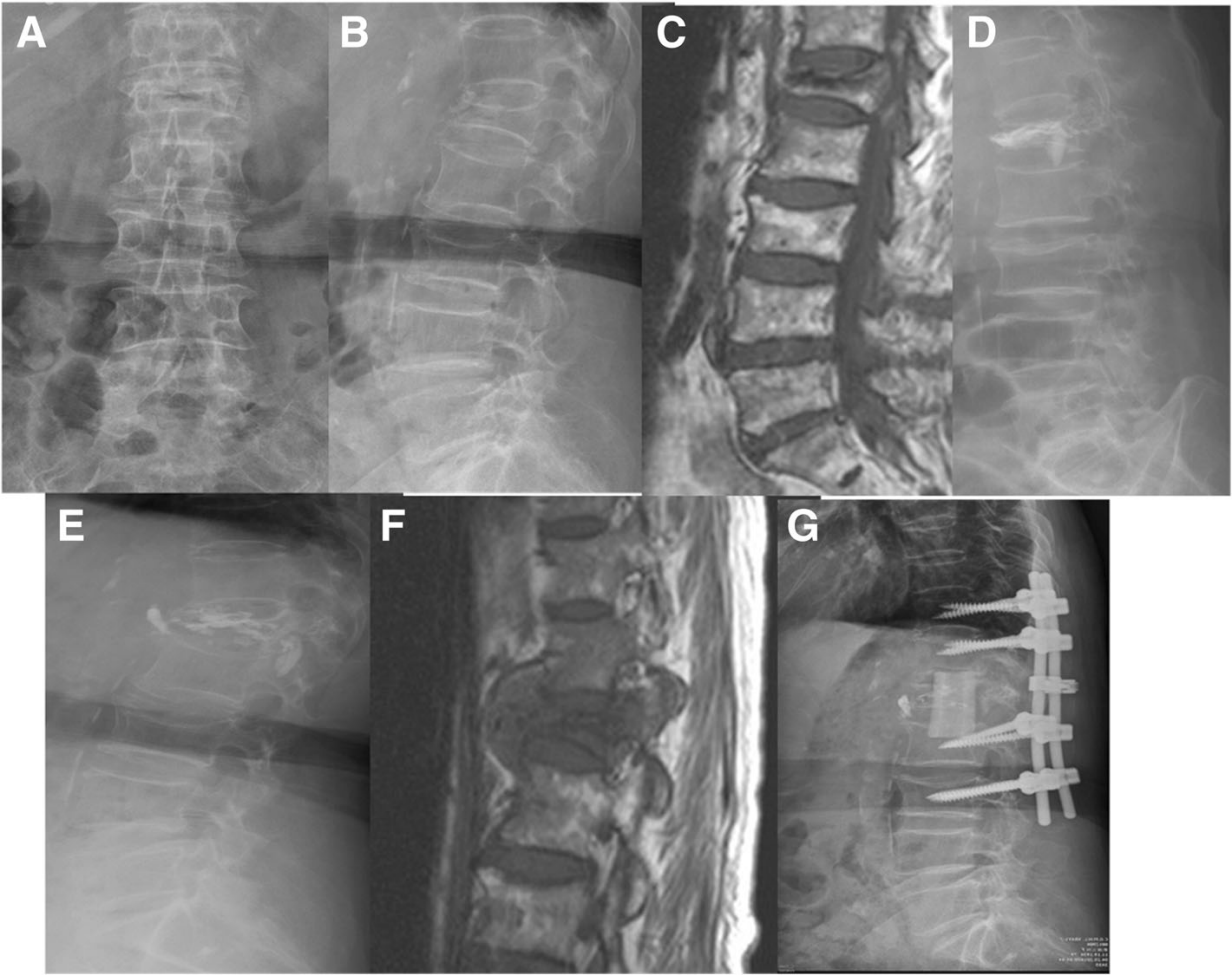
A case represented tuberculosis infection after vertebroplasty (VP). Initial radiographic images and MRI revealed L1 compression fracture (a, b, c). This patient received L1 VP first (d). After VP, back pain was subsided dramatically, however, severe low back pain without neurologic deficits was appeared again four months later. Plain radiograph showed L1 body collapse with cement breakage (e). MRI revealed L1 spondylitis with abscess formation (f). Reconstruction revision surgery was performed for this patient (g). Tissue culture showed mycobacterium tuberculosis
